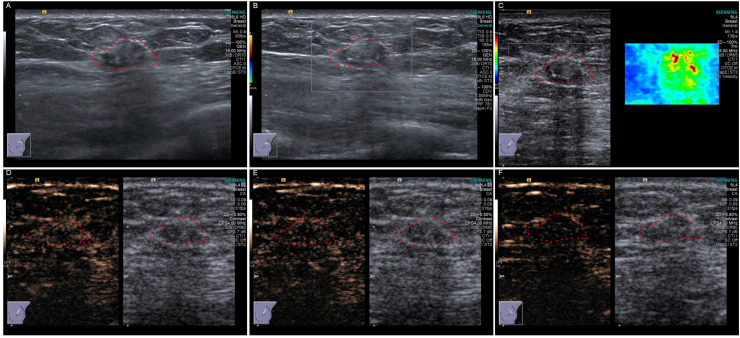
Multimodal ultrasonographic manifestations of Case 13 (breast JXG): (A) B-mode ultrasound showed an irregular hypoechoic mass with ill-defined margins and internal calcification. (B) Color Doppler flow imaging revealed no significant internal and peripheral vascularity. (C) Shear wave elastography depicted tissue stiffness characteristics: based on the color scale of elastic modulus, the image showed that the interior of the lesion was predominantly orange and red signals, which form a clear contrast with the surrounding normal glandular tissue (which appears blue or green, indicating a soft texture). Quantitative analysis results indicated that the average shear wave velocity of the lesion was 6.84 m/s, much higher than that of the adjacent glandular tissue. This hardness manifestation conforms to the imaging features of a solid, relatively hard lesion in the BI-RADS classification, suggesting that the lesion has a relatively high tissue hardness and a higher possibility of malignancy. (D–F) Contrast-enhanced ultrasound sequences showing dynamic perfusion patterns in the arterial phase (25 s) (D), venous phase (40 s) (E), and delayed phase (120 s) (F). CEUS revealed slight homogeneous enhancement. The area delineated by the red dashed circle represents the tumor region.
